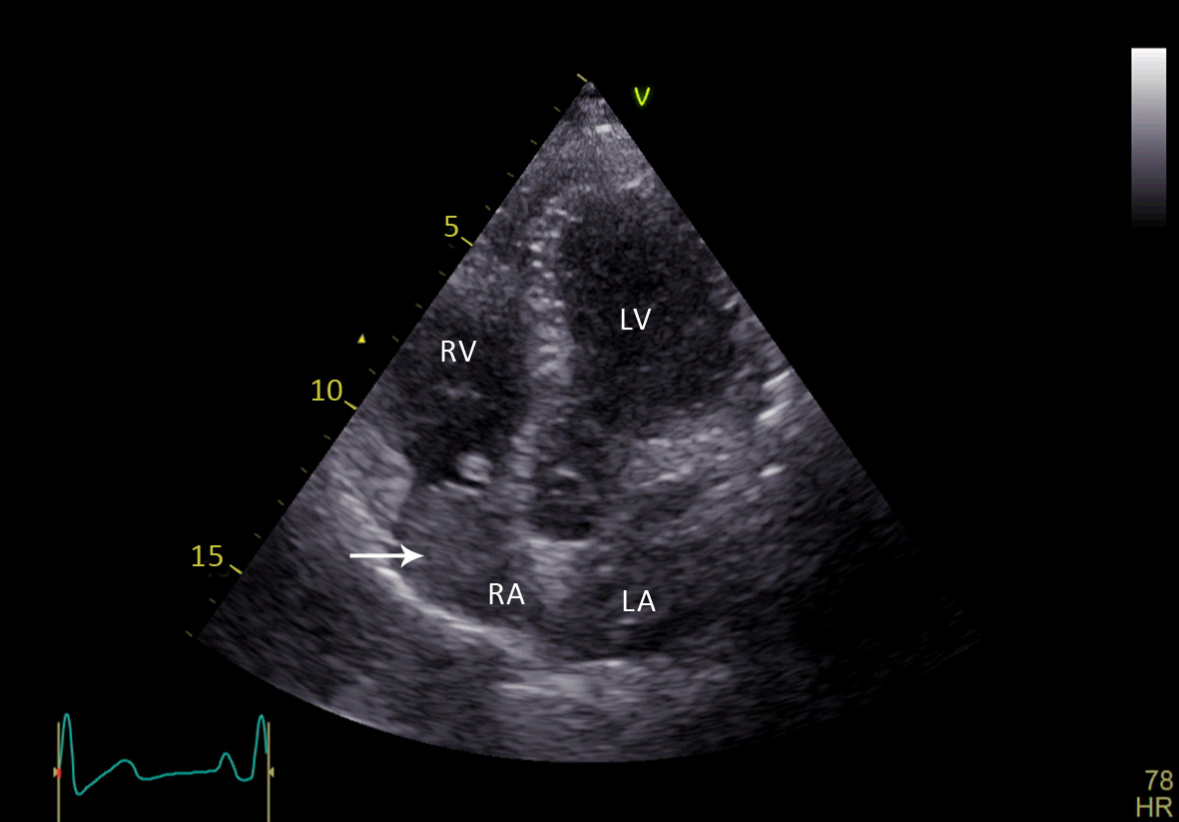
Transthoracic echocardiography (apical 4-chamber view) shows a fixed right atrial nonhomogeneous mass, which is attached to the roof of the right atrium.
